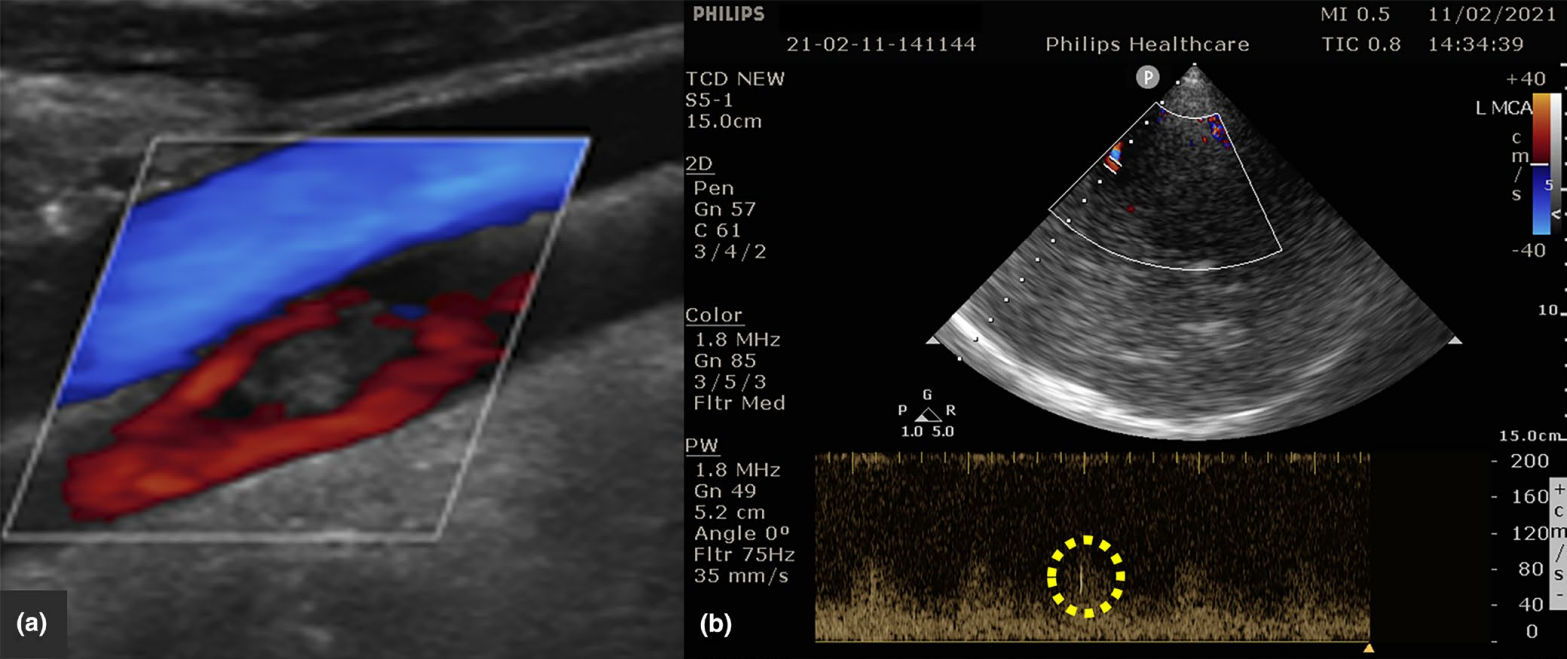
Patient with acute ischemic stroke due to floating thrombus in the left internal carotid artery (a) with positive microembolic detection on the ipsilateral middle cerebral artery as detected by Transcranial Color‐Coded Doppler (b).
